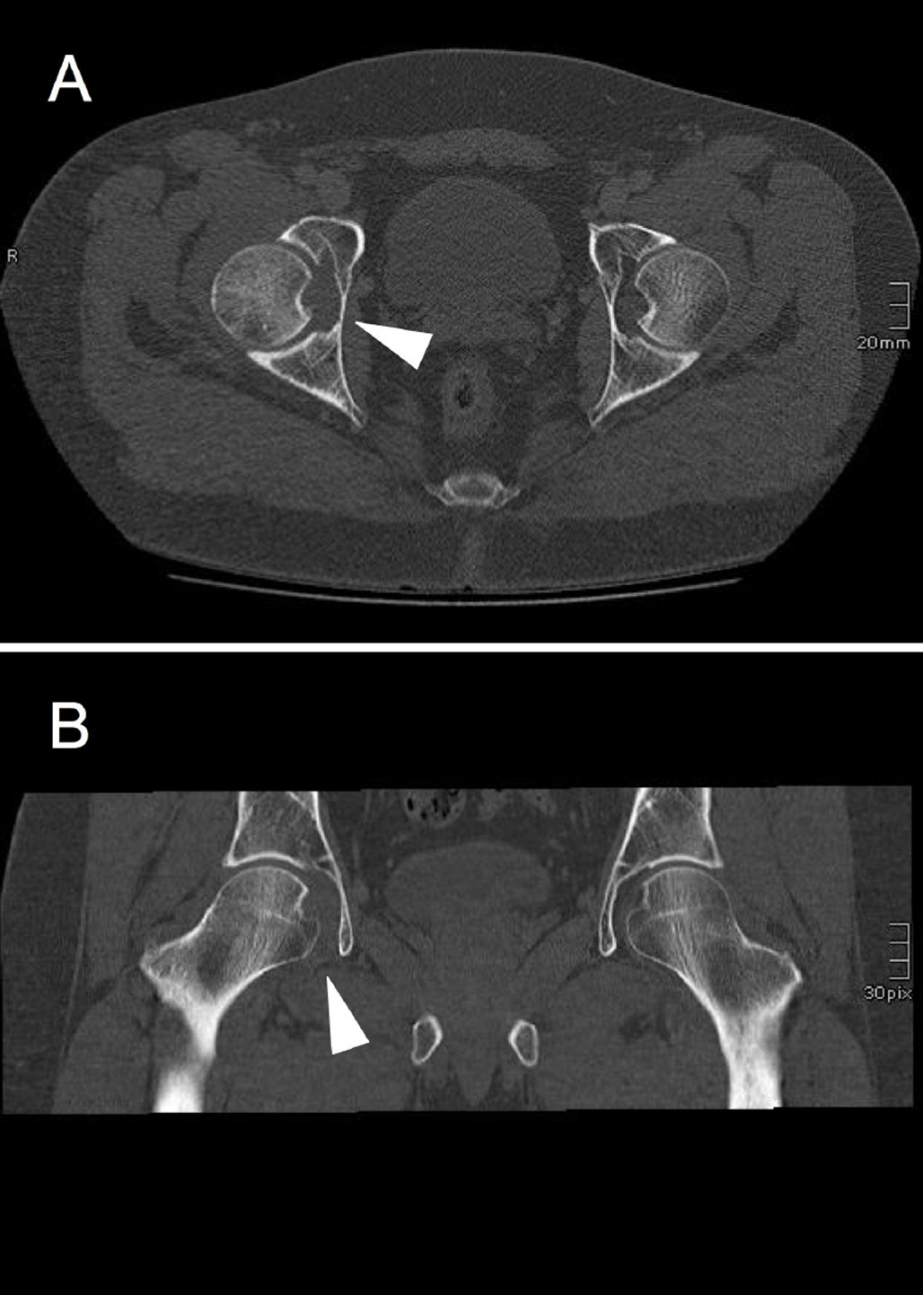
Un-enhanced thin-slice CT of the hips in transverse-oriented source images (A) and coronal reformats (B). The arrows show the soft tissue-isodense mass, which filled up the right joint space and led to a widening of the joint space compared to the contralateral side. No calcifications or massive extrinsic bone erosions could be seen.
